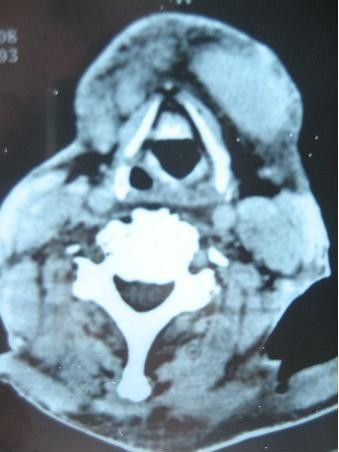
Computed tomography scan showing the upper glottis area free of any tumor.
